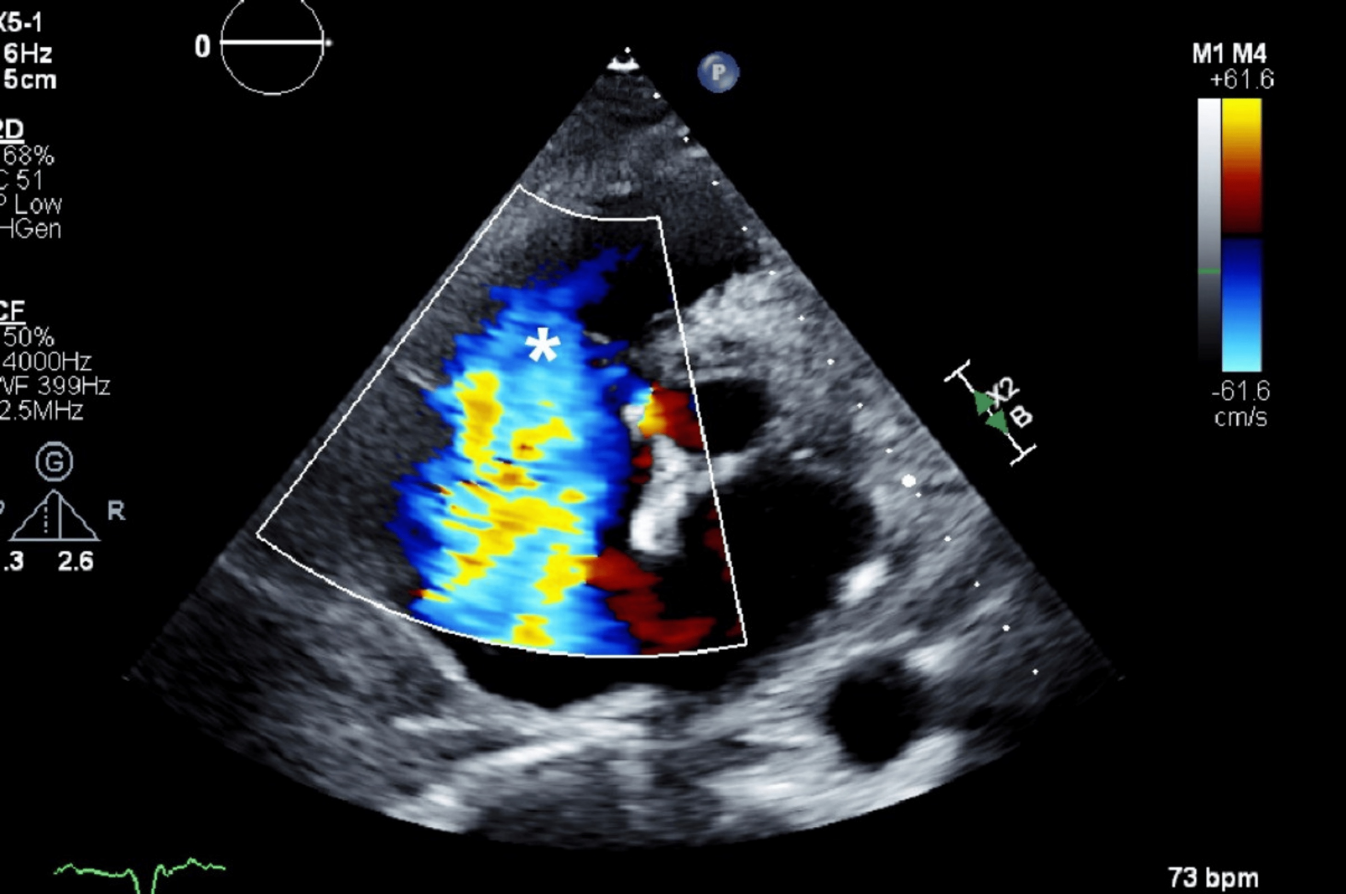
Transesophageal short-axis cut showing the huge central jet (asterisk) of severe tricuspid regurgitation
